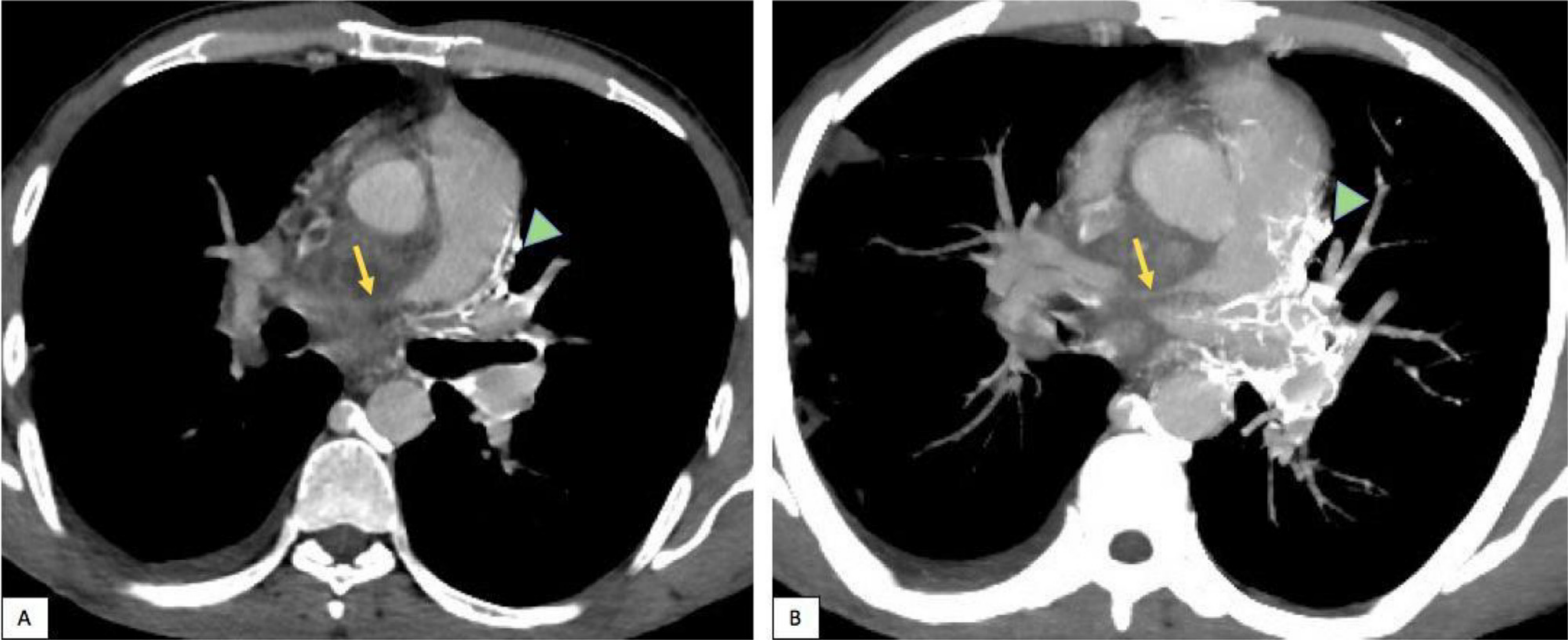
Axial contrast-enhanced CT images (soft-tissue window, before (A) then after MIP reconstruction (B), showing encasement and intense narrowing of the right pulmonary artery (arrows), with left mediastinal collateral channels (arrowhead). We note also a small thrombus in the lumen of the proximal right atrium (A).
